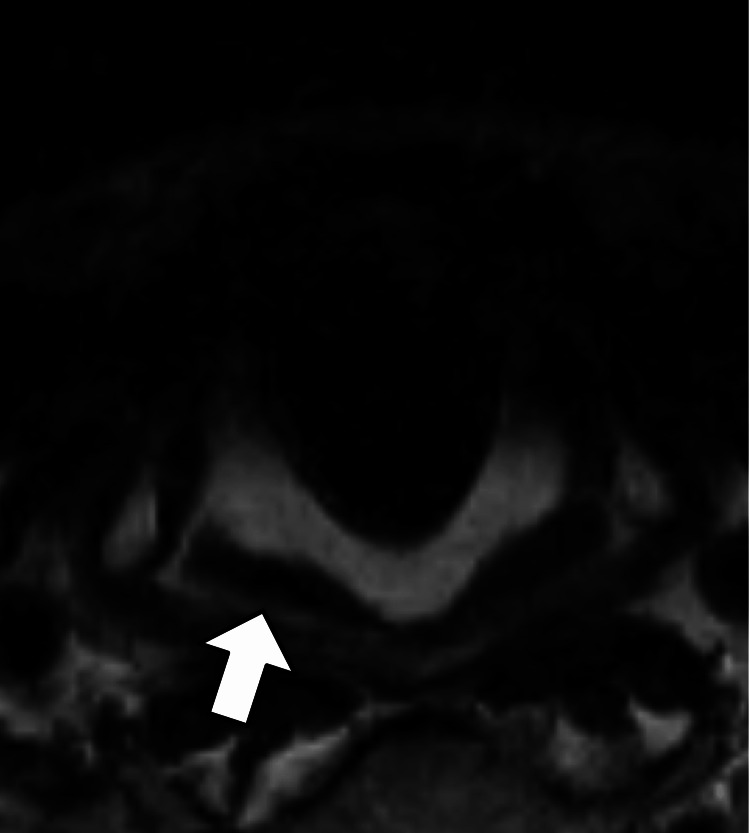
Laryngeal MRI finding Axial T2-weighted MRI on initial admission shows no atrophy of the posterior cricoarytenoid (PCA) muscle (arrow).
